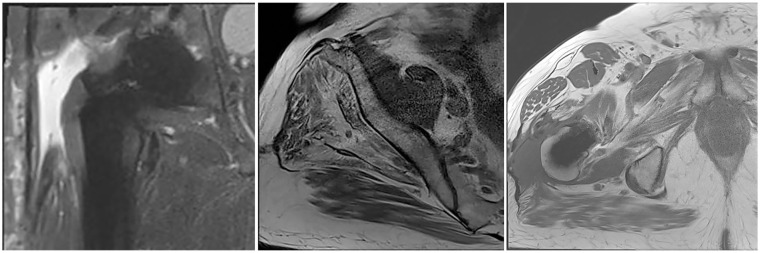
MR images depicting a complete avulsion of the gluteus medius and minimus tendon as well as a fatty degeneration of both muscles.
